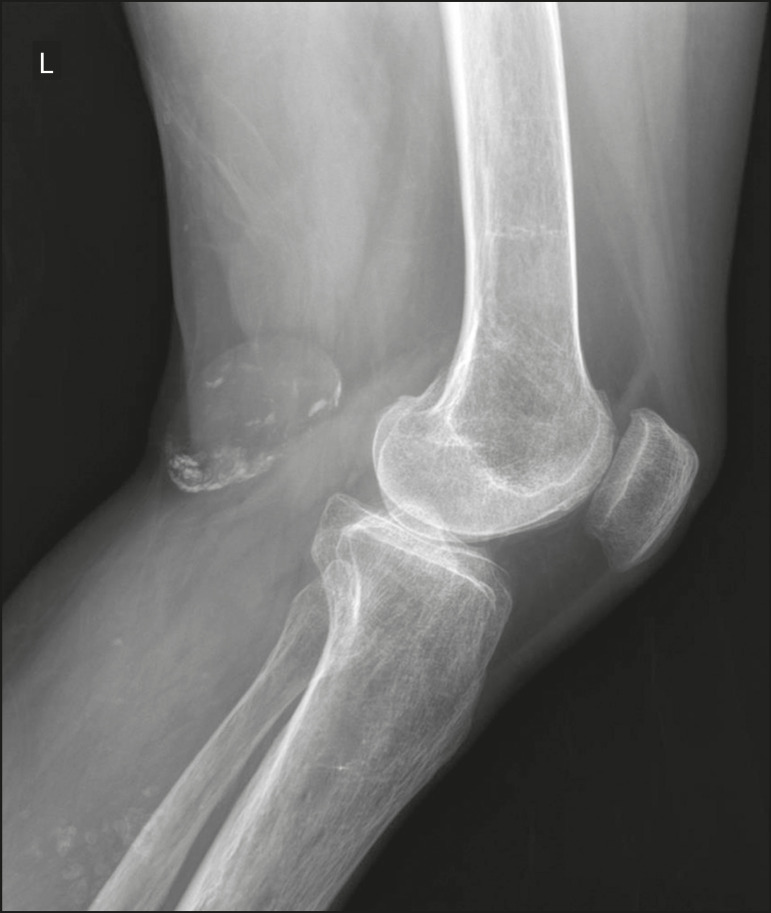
Popliteal artery aneurysm. Lateral X-ray of the knee showing peripheral oval-shaped calcifications along the course of the popliteal artery, which presents dilation consistent with an aneurysm.
